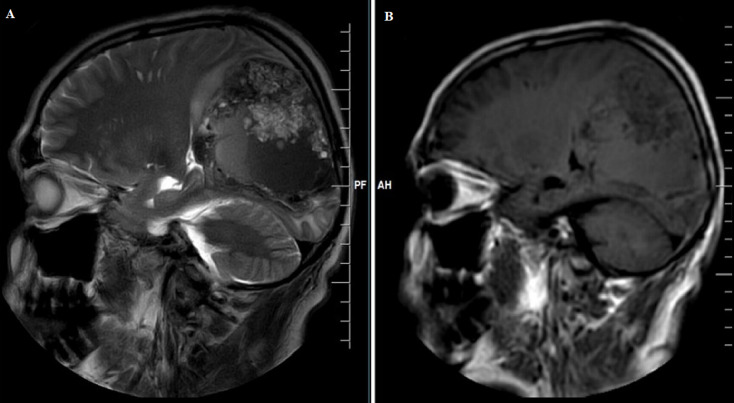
A,B) MRI of the brain showing multiple areas of blooming in right parieto-occipital region on susceptibility weighted imaging (SWI)
